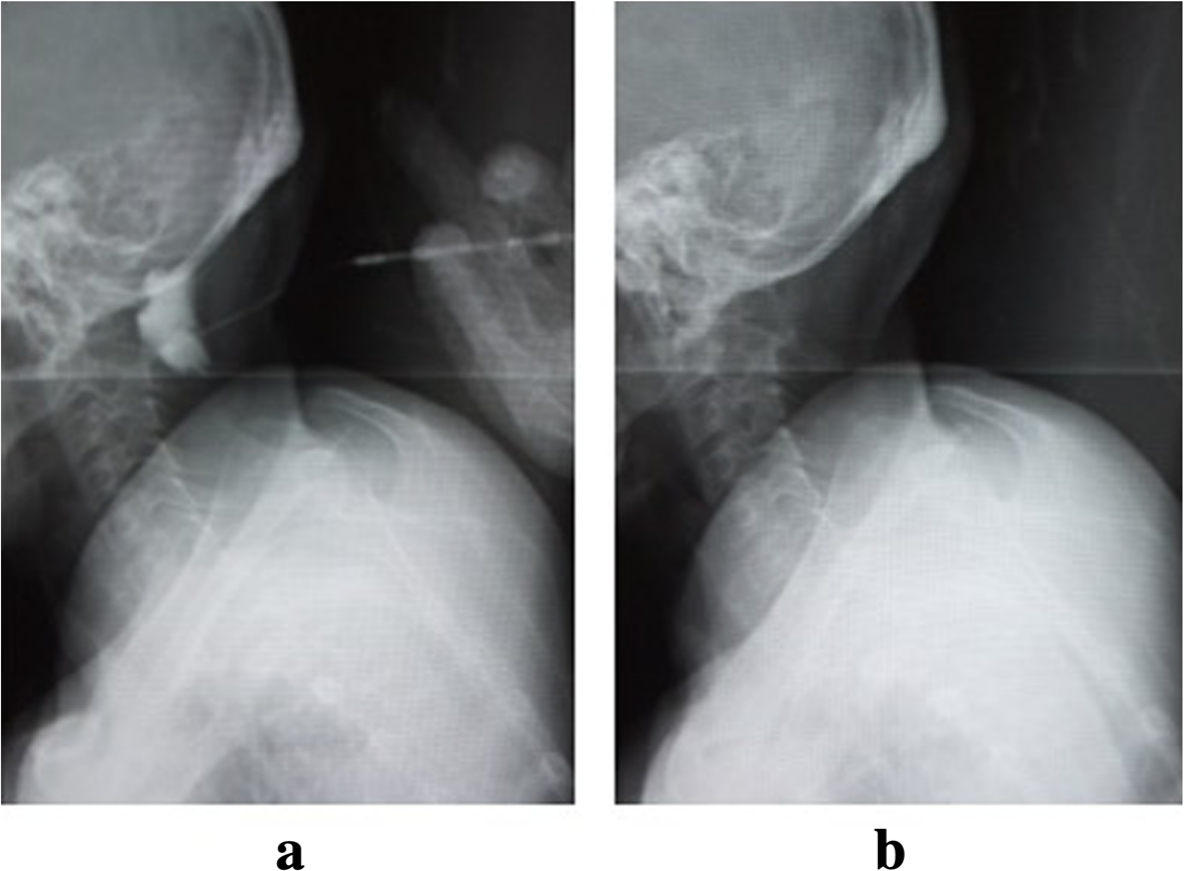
X-ray taken during the aspiration of the pseudomeningocele. a X-ray taken of the pseudomeningocele during the injection of contrast medium. b X-ray taken after aspiration of the contrast medium and spinal fluid
